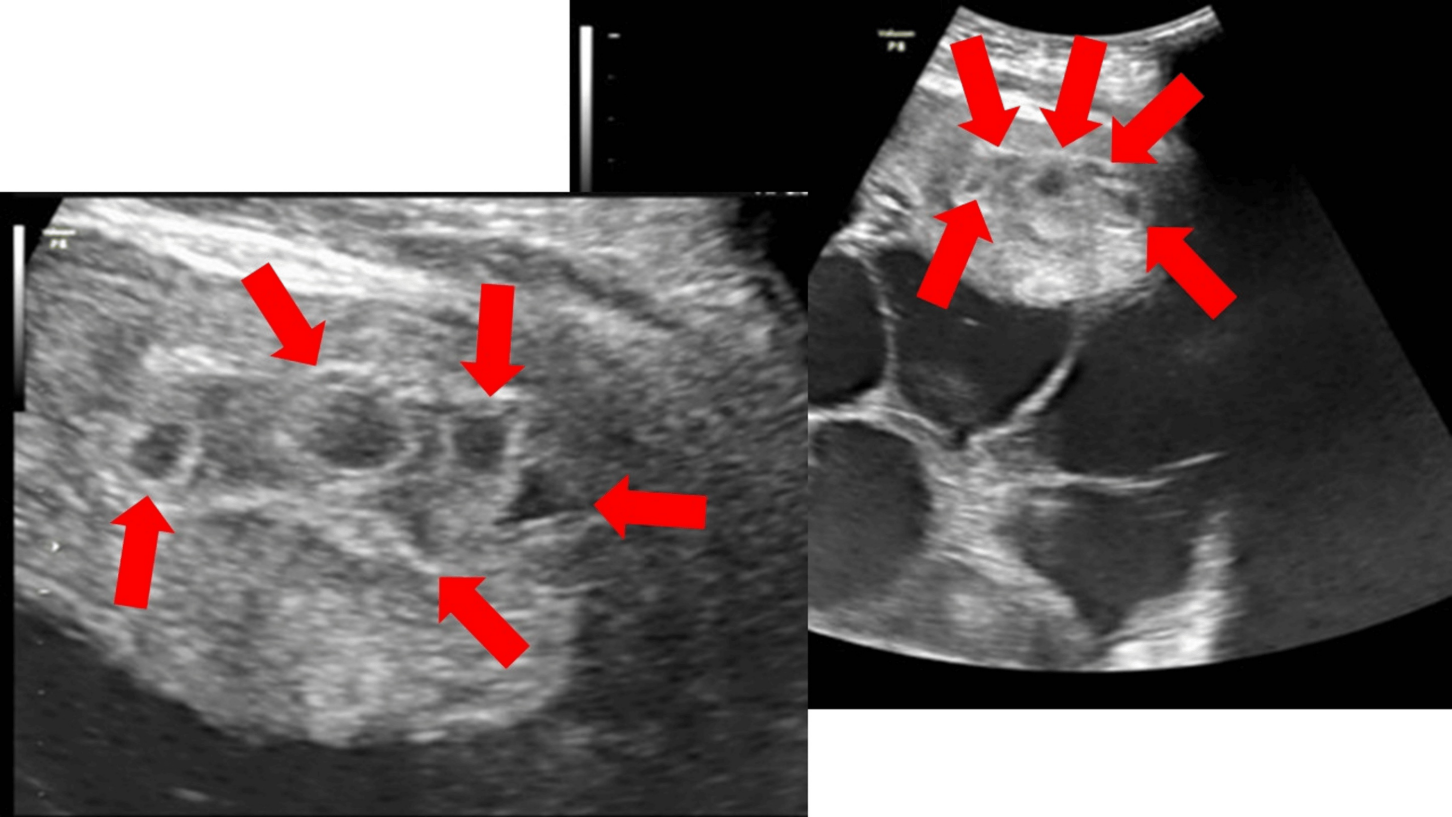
Transabdominal sonogram showing five gestational sacs within the uterine cavity at the time of diagnosis of a quintuplet pregnancy
